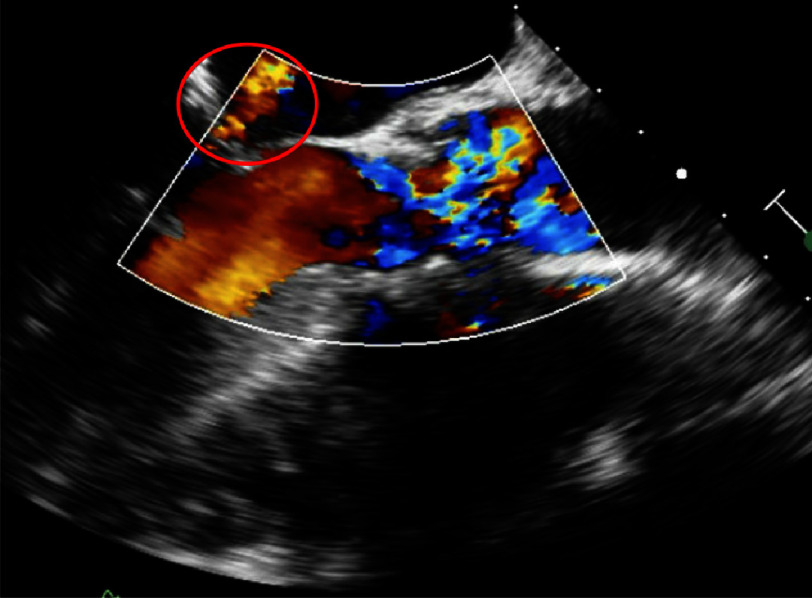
Transesophageal echocardiography demonstrating a small peri membranous ventricular septal defect with trivial left to right shunting by color flow Doppler.
